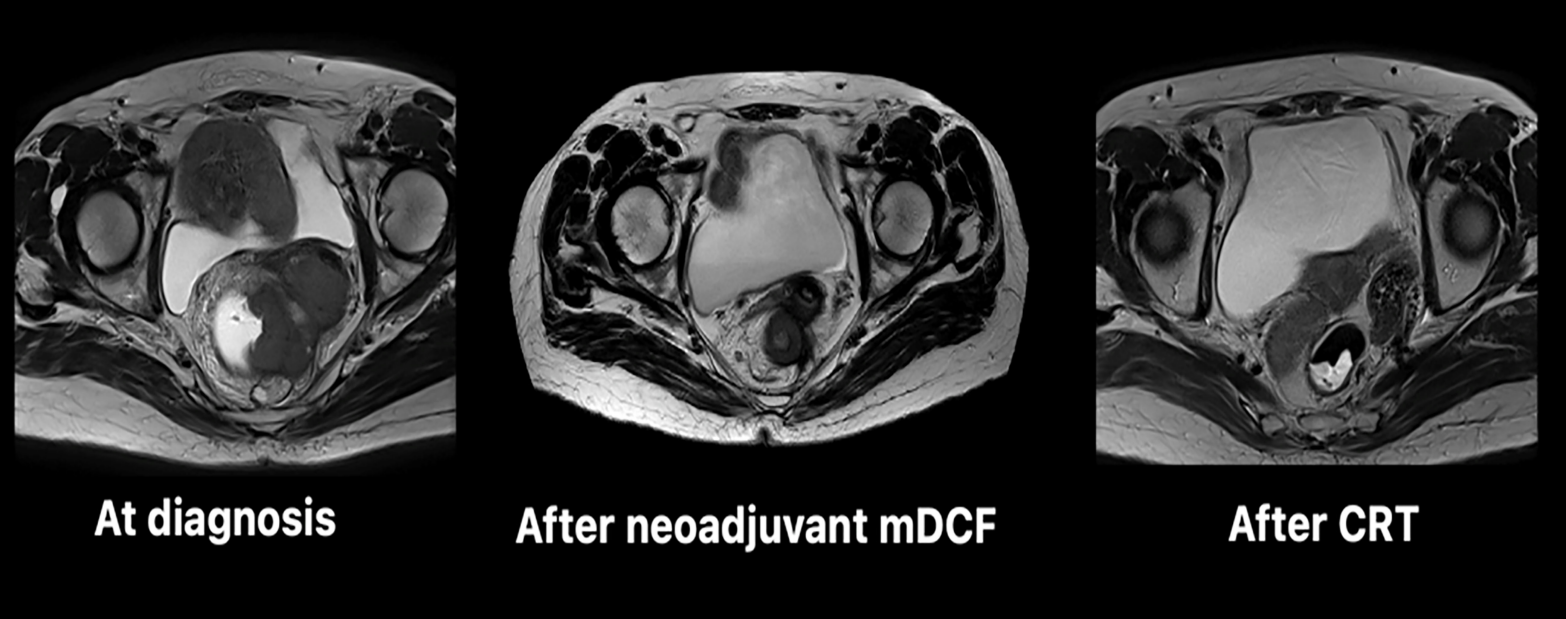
Evolution of the rectal squamous cell carcinoma followed by pelvic MRI during treatment (patient #1). CRT, chemoradiotherapy; mDCF, modified DCF chemotherapy.
